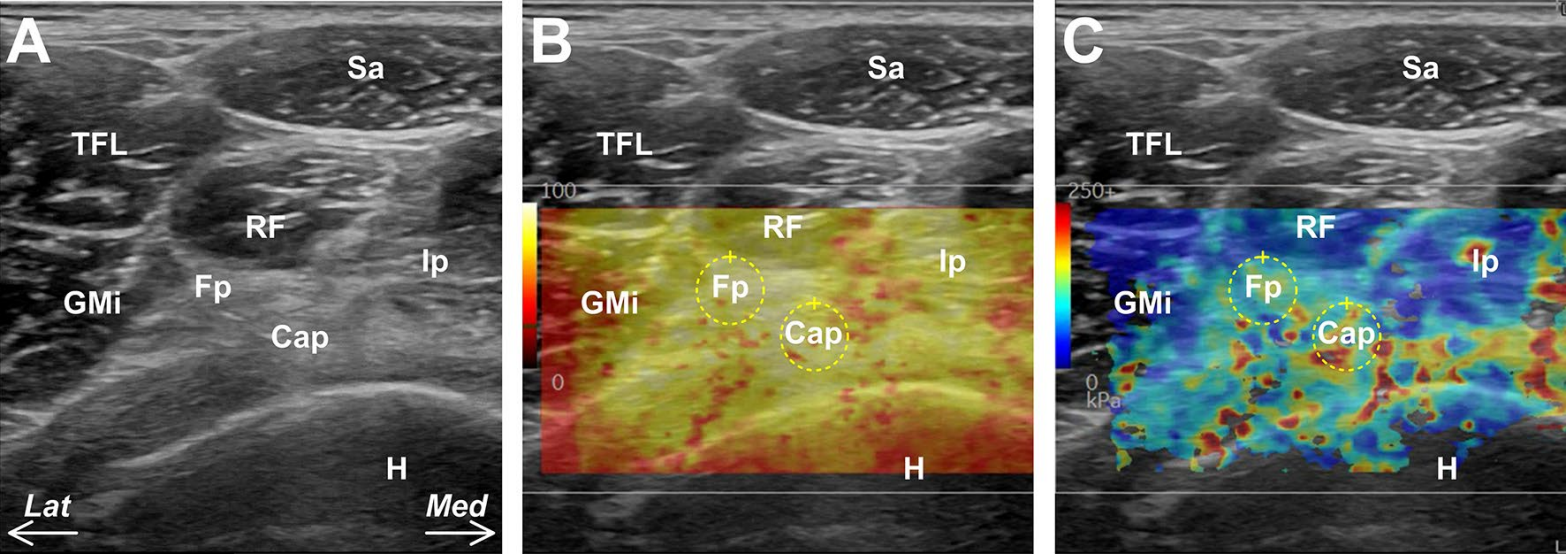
Ultrasonographic images of the anterior region of the joint capsule. (A) Short-axis image at the proximal level of the femur head (H). The fat pad (FP) is located deep to the rectus femoris (RF), medial to the gluteus minimus (GMi), lateral to the iliopsoas (Ip), and superficial to the joint capsule (Cap). (B) Quality map of the shear wave. Yellow and red represent shear waves with high and low qualities, respectively. (C) Shear-wave elastographic images. The red and bright colors represent high shear wave velocity, and blue and dark colors represent low shear wave velocity. *Sa* sartorius, *TFL* tensor fasciae latae; Yellow circles: measurement points of the shear wave velocity; *Lat* lateral, *Med* medial.
